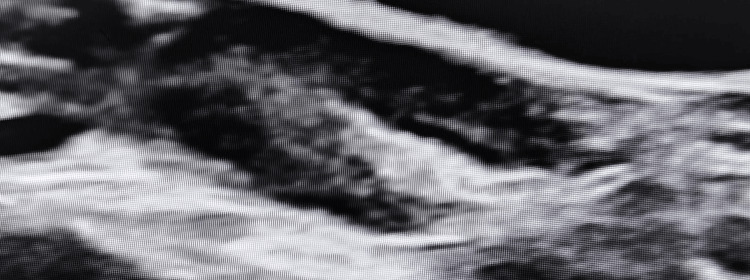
Ultrasonography of the axillary lymph node
